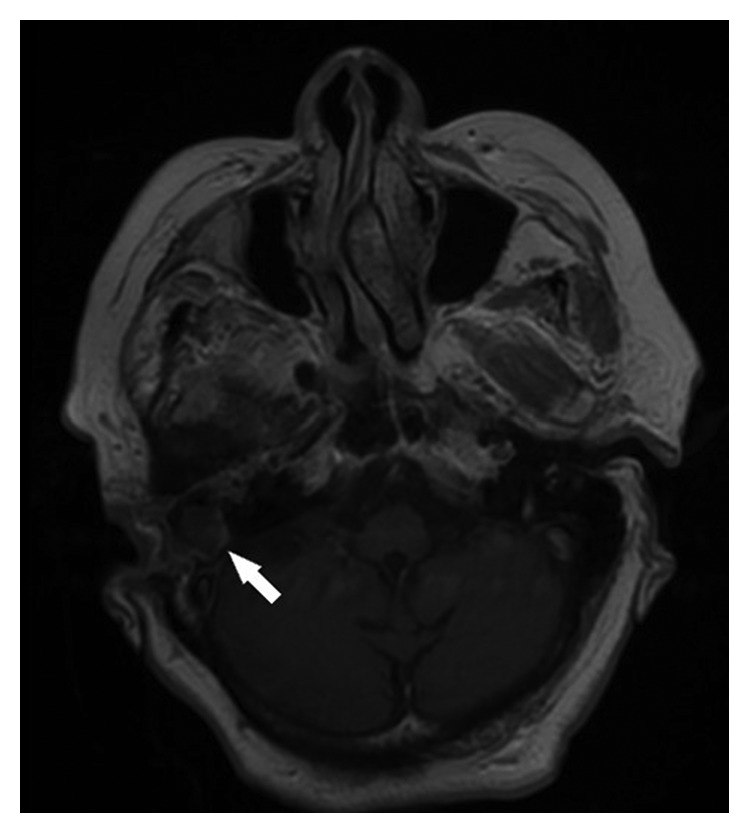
Contrast-enhanced ear MRI axial section. Approximately 2 cm mass showing peripheral contrast enhancement in the right mastoid antrum (white arrow).
